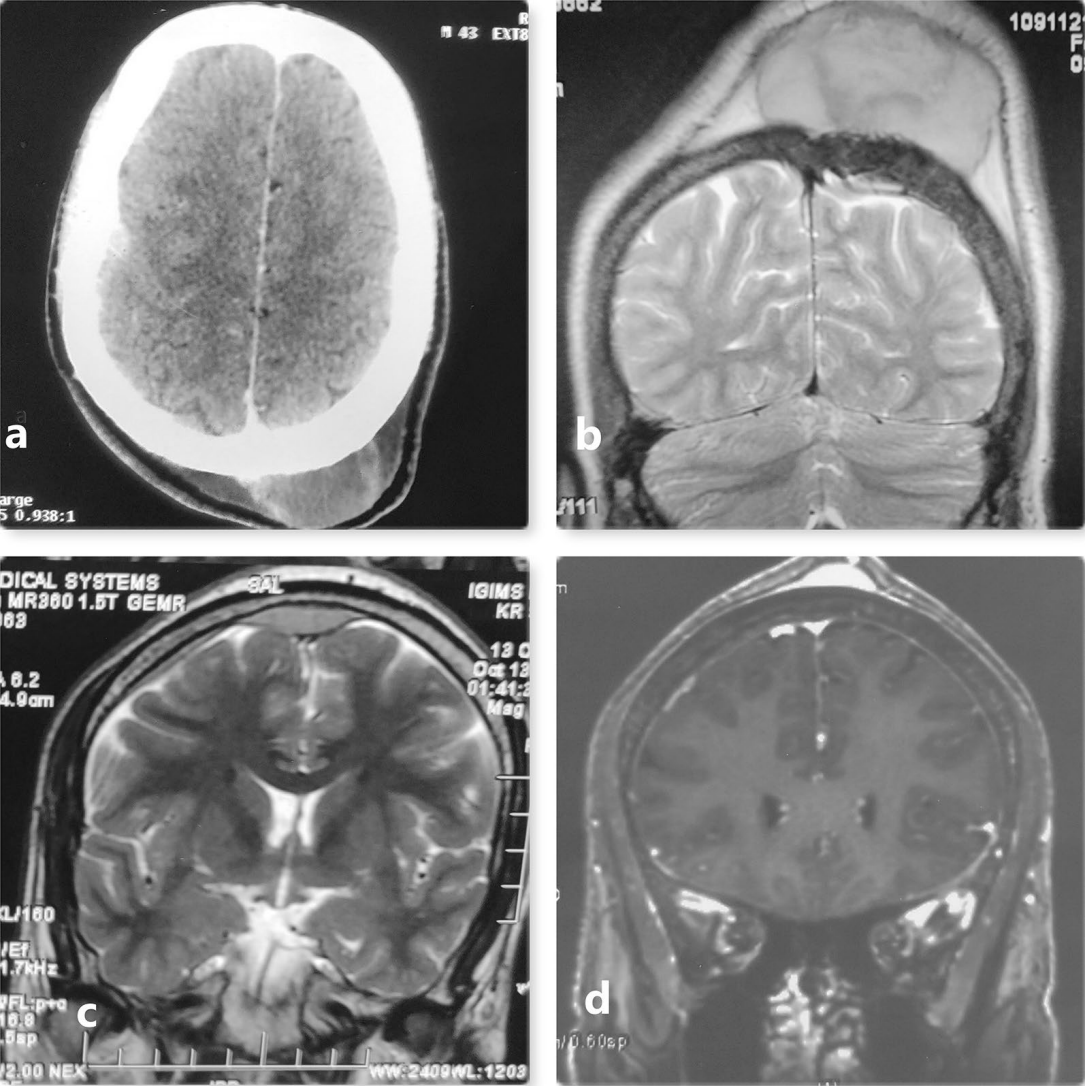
a Nonenhanced computerised tomography scan suggesting lesion of size 3 cm without underlying cortical changes in calvaria. lesion is isodense. b On Coronal T2Magnetic resonance imaging (MRI) images done 6 months later shows increase in size of the lesion to around 10 cm with new lesion in with cortical changes in underlying bone suggesting selective permeation of tumour tissue. c Coronal T2 weighted image suggestive of lesion in midline with involvement of anterior sagittal sinus and left parietal region Dural involvement. d Coronal contrast MRI suggesting homogenous contrast enhancement of the lesion with Dural tail sign present
